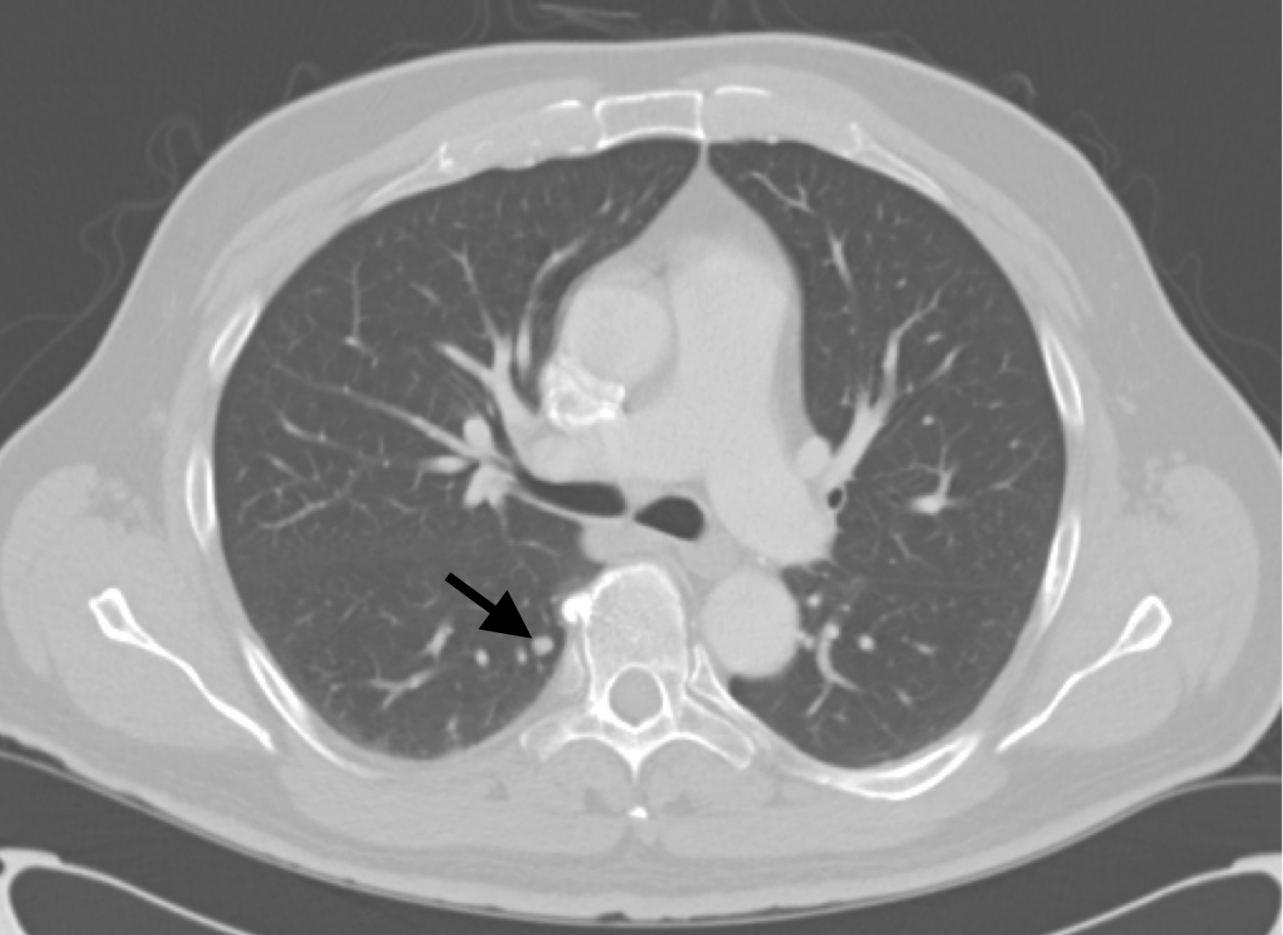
July 2017 CT chest with IV contrast showing a 6-mm lung nodule (arrow) CT: computed tomography; IV: intravenous
